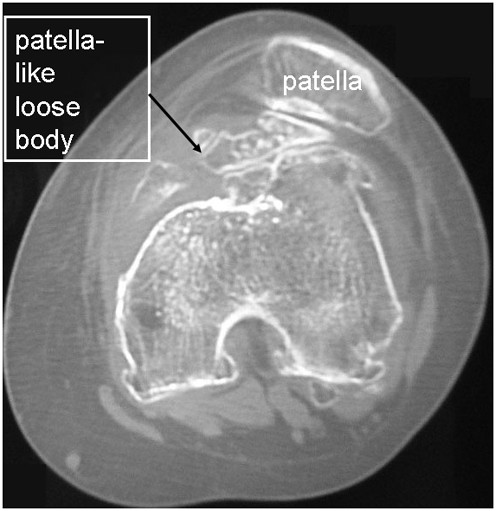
CT. The left knee had formed a double-layered patella.
